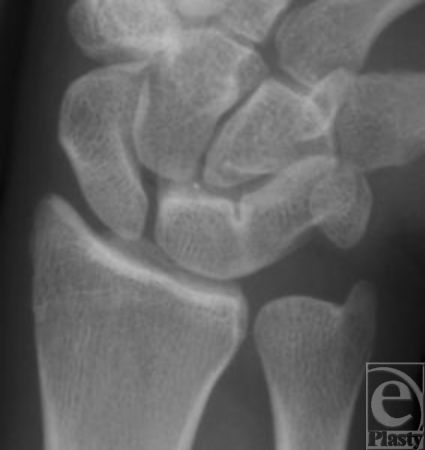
Anteroposterior radiograph of the right painful wrist showing a luno-triquetral coalition Minaar type II.
